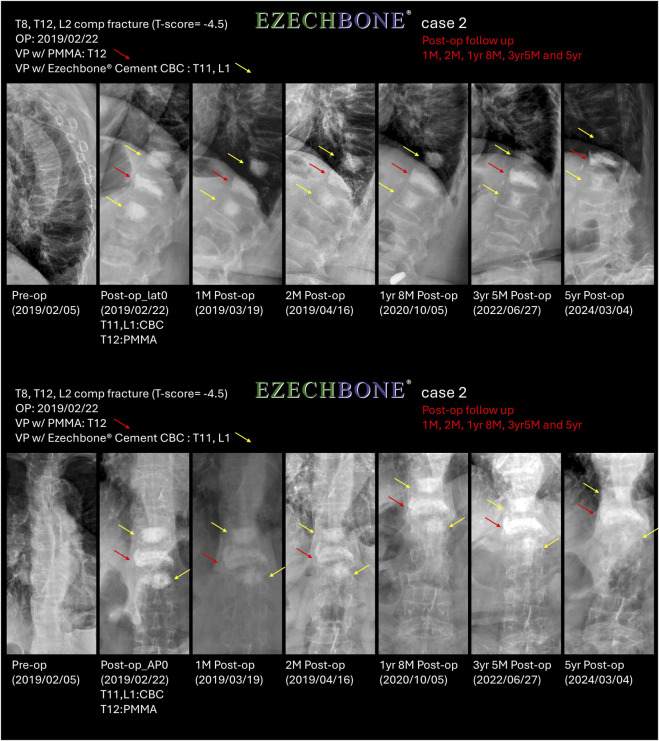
The preoperative and a series of follow-up radiographs in case 2.
